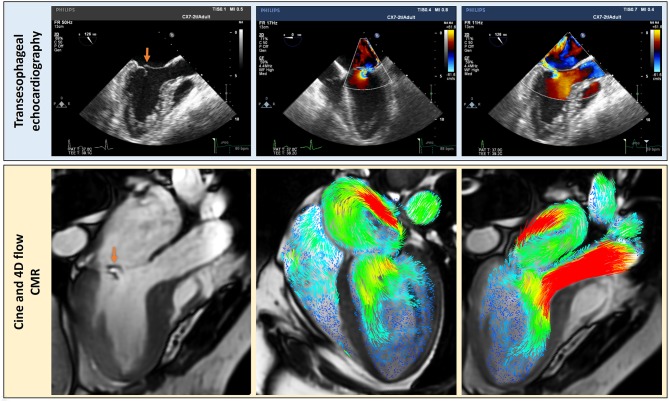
Complementary clinically relevant details can be assessed by using echocardiography; transesophageal echocardiography in this case (blue panel) and CMR with 4D flow streamline visualization. The MR flow convergence can be better visualized by echocardiography but 4D flow CMR is better to visualize MR jet within the left atrium. Hence, even though traditional methods of PISA and vena contracta have been proposed for 4D flow CMR, the current best method appears to be the direct MR jet quantification in the left atrium using the retrospective valve tracking method.
